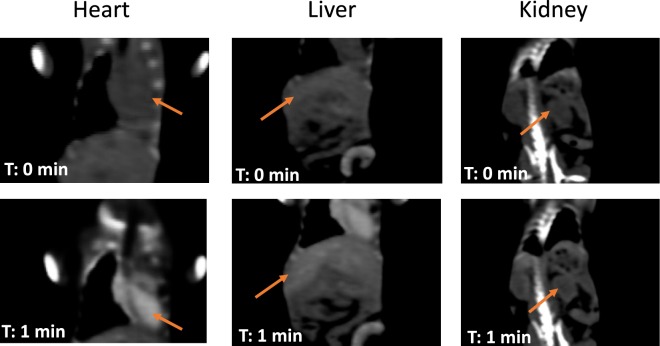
CT images at T = 0 and 1 minute.
